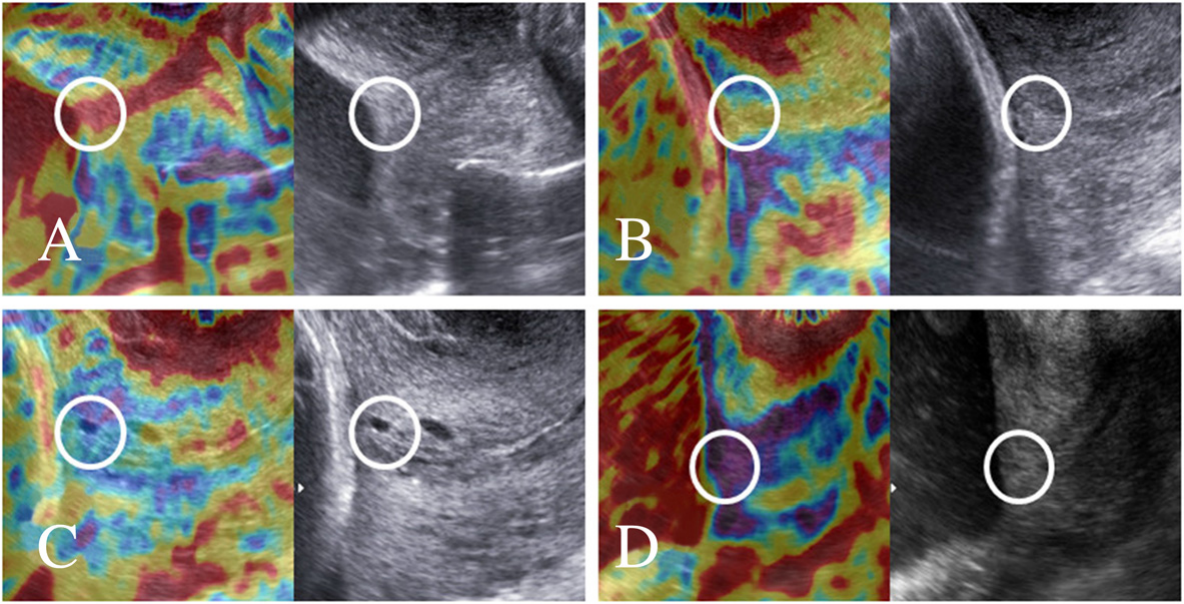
Elastographic (left) and ultrasound (right) images of the internal cervical os (circles) at 18-22 weeks of pregnancy. Internal cervical os stiffness was assessed as: A) soft (red); B) medium soft (yellow); C) medium hard (blue); D) hard (purple).
